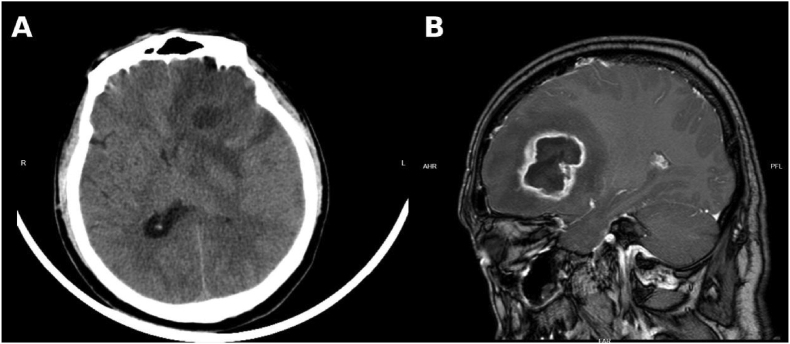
Neuroimaging findings on Day 49 (D49). (A) Brain CT obtained on Day 49 (D49) demonstrating a space-occupying lesion in the left frontal lobe with surrounding edema. (B) Contrast-enhanced magnetic resonance imaging (MRI) revealing a ring-enhancing lesion consistent with a brain abscess.
